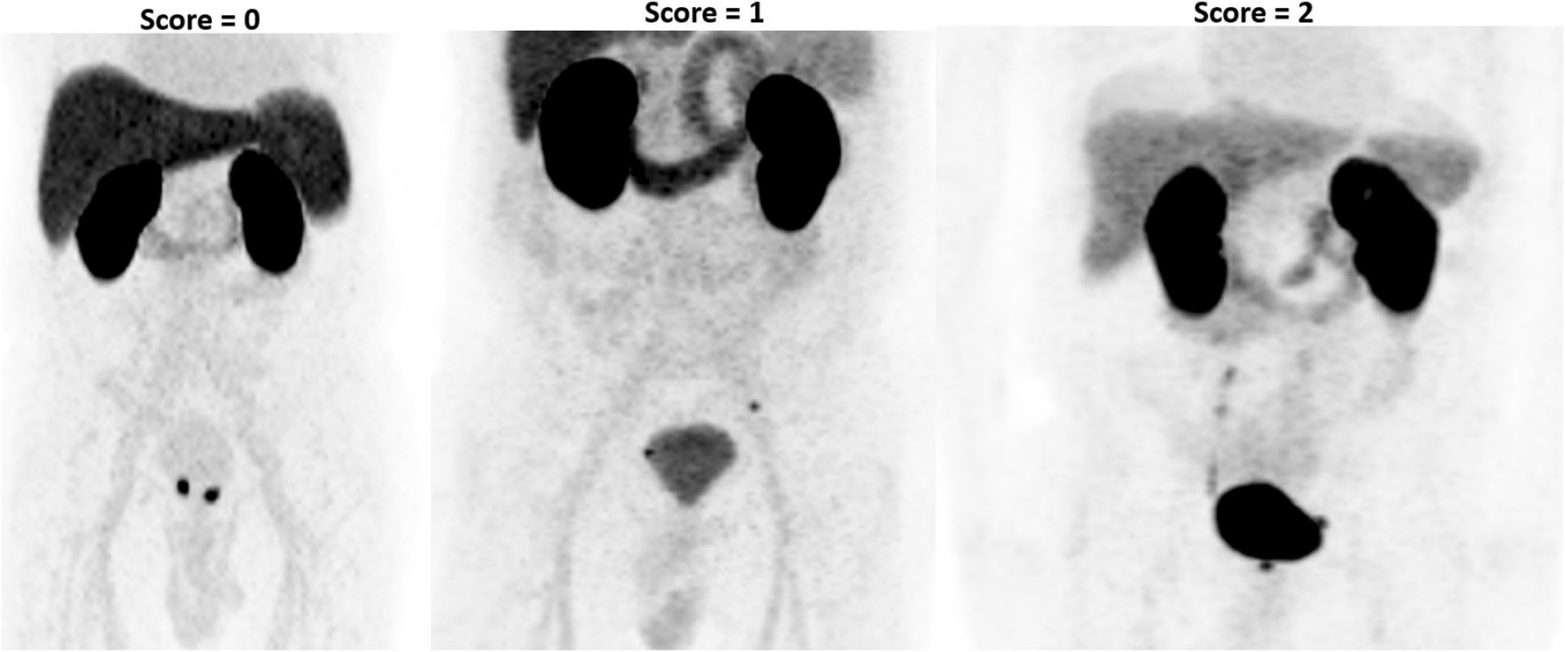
Representative scoring in the qualitative bladder activity analysis. Maximum intensity projection images captured at SUV 0–10
